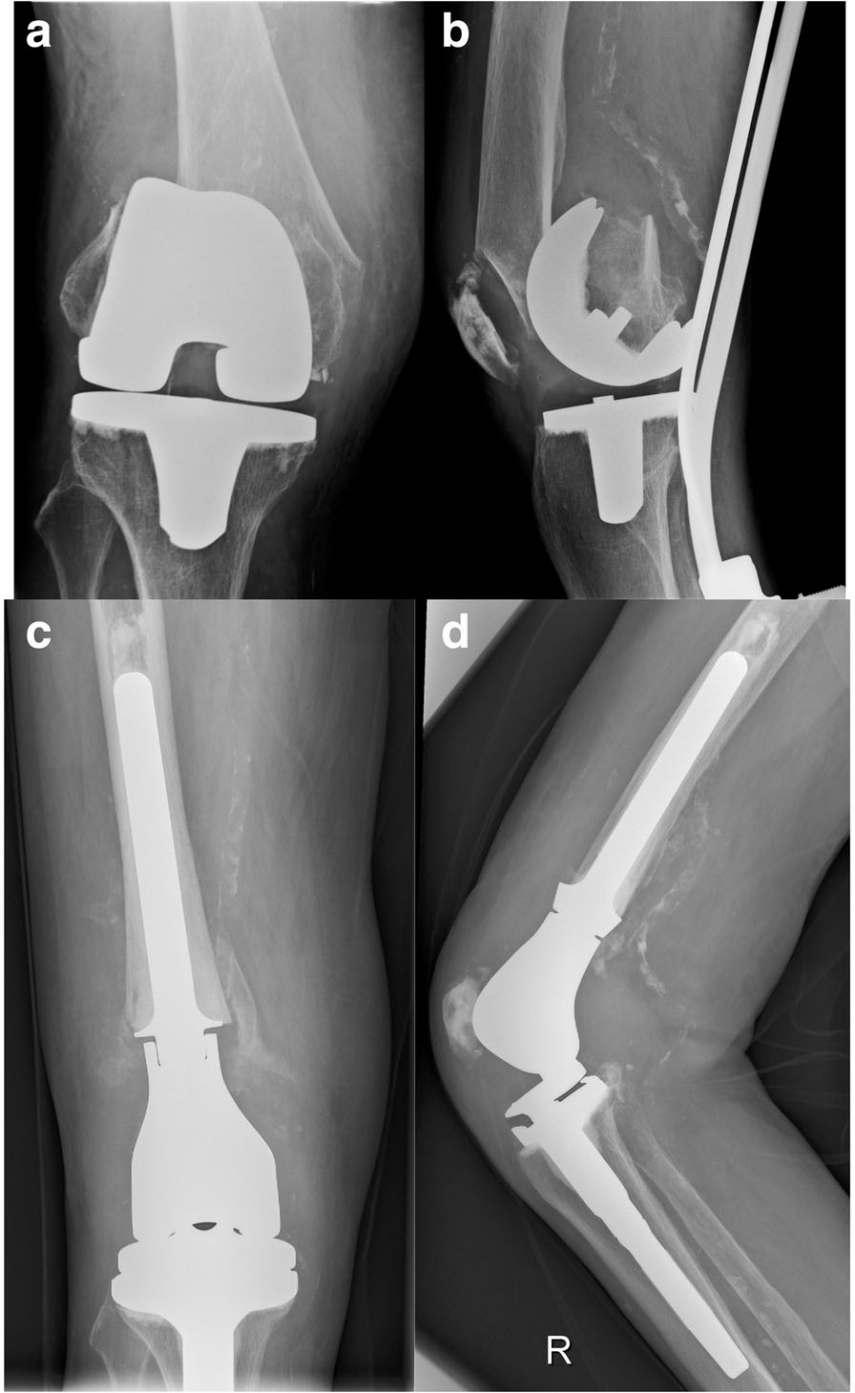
a, b Anteroposterior and lateral radiographs of an 81-year old female patient with severe periprosthetic fracture of the distal femur after trauma; c, d Anteroposterior and lateral radiograph of the same patient 4 months after consecutive reconstruction of the distal femur
